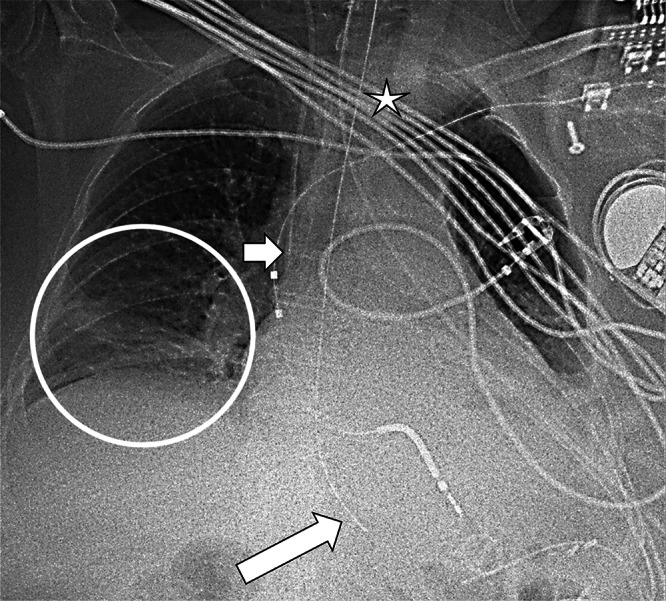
Chest radiography shows multiple supportive devices (star = endotracheal tube; short arrow = jugular central venous catheter; long arrow = nasogastric tube, NGT) and a lung consolidation in the right lower lobe (circle). The tip of the NGT projects under the diaphragm.
